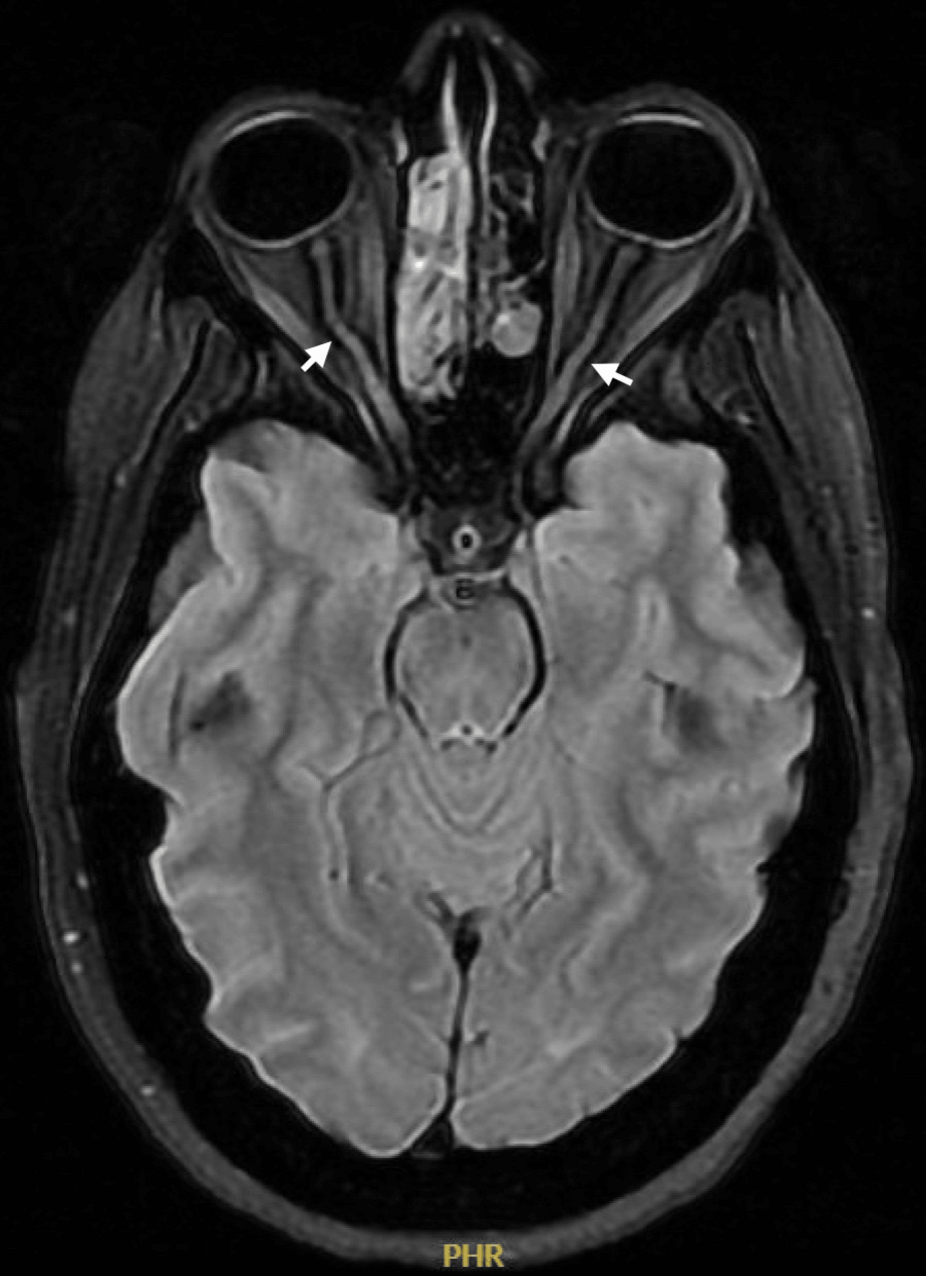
MRI (axial view) T2-weighted fat suppression showing bilateral tortuous optic nerve (arrows).
